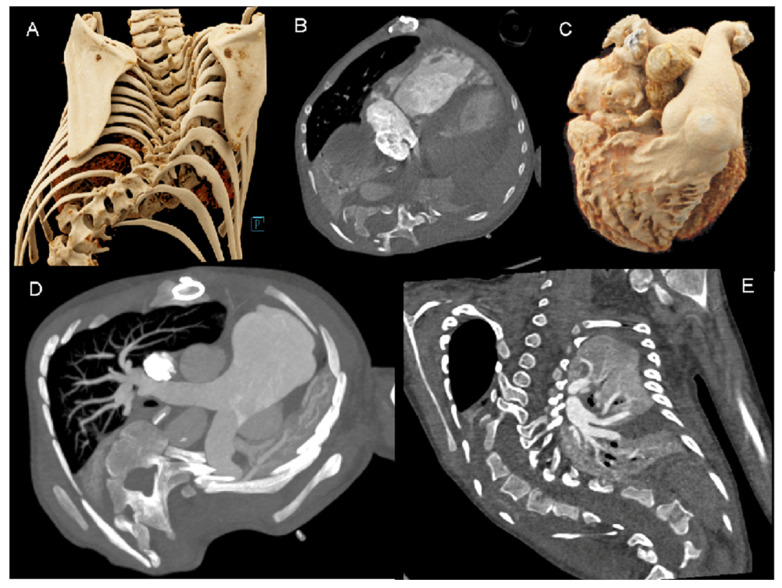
Computed tomography angiography (CTA) of the chest. (A) 3D cinematic volume rendering reconstruction (VRT) of the thoracic skeleton (posterior view) demonstrating severe thoracic rotoscoliosis and thoracic cage deformity. (B) Axial view demonstrating pectus carinatum. (C) 3D cinematic volume rendering reconstruction (VRT) of the heart (anterior view) and (D) axial view demonstrating severe dilation of the pulmonary artery. (E) Coronal view demonstrating severe rotoscoliosis and atelectasis of the left lung with mild pleural effusion.
